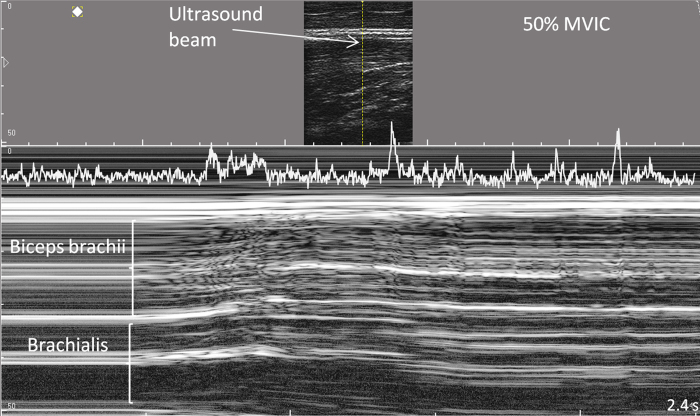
M-mode ultrasound trace of the biceps brachii and brachialis muscles during isometric elbow flexion at 50% of the maximal force. Note the gradually increasing motion that starts in the deeper muscle regions. The TKEO-transformation of the greyscale values of the upper cm of the biceps brachii is projected into the top of the trace.
